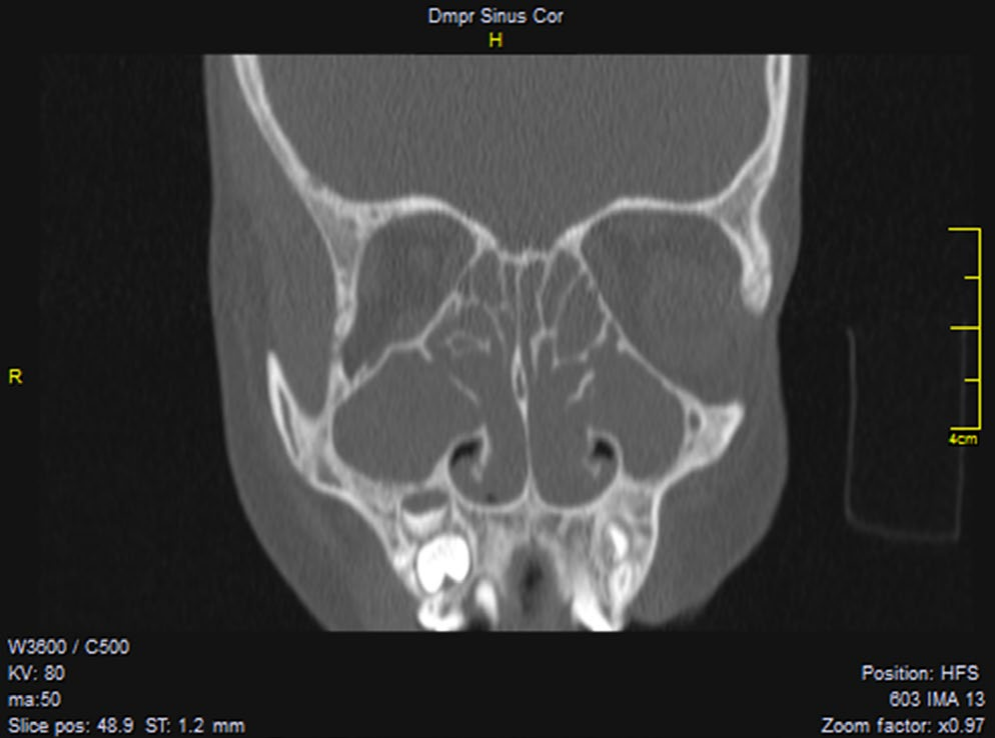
Sinus CT scan. Coronal view of sinus CT scan showing opacities of all sinuses with bilateral medial bulging of the nasal lateral walls
